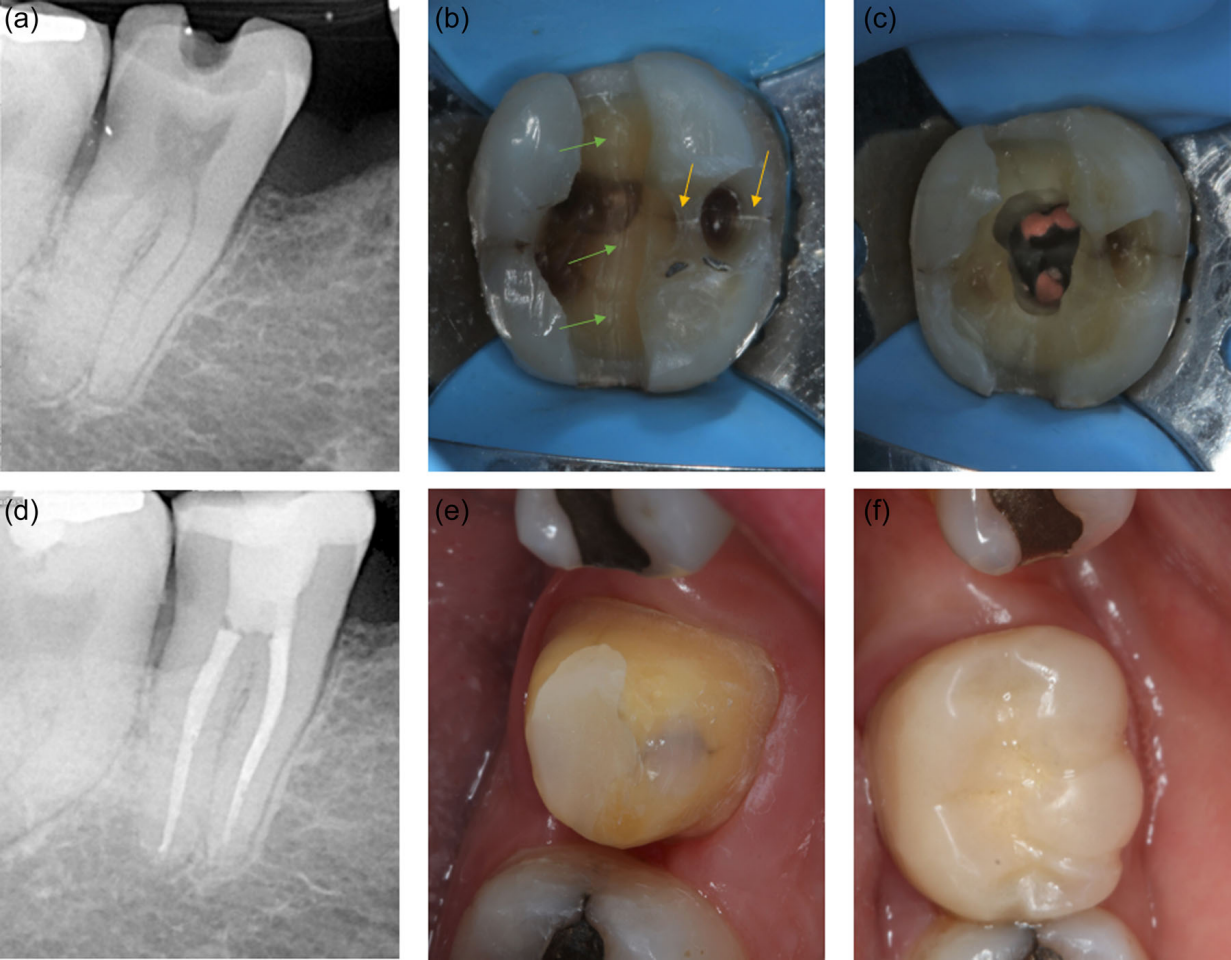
Treatment sequence of a cracked 47 with irreversible pulpitis (a) preoperative long‐cone periapical radiograph (b) removal of previous amalgam restoration revealing multiple crack lines running towards mesiodistal (green arrows) and buccolingual direction (yellow arrows) (c) root canal obturation completed (d) periapical radiograph after completion of endodontic treatment (e) crown preparation (f) occlusal view after cementation of a metal ceramic crown.
